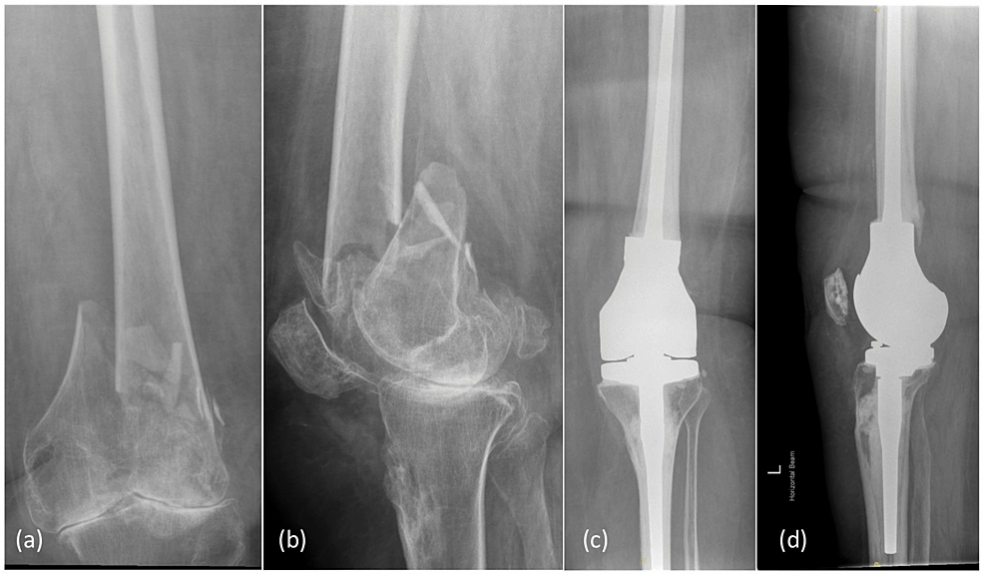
A 73-year-old lady with a distal femur comminuted fracture treated with distal femur replacement Preop xrays (a,b), Postop xrays (c,d)
